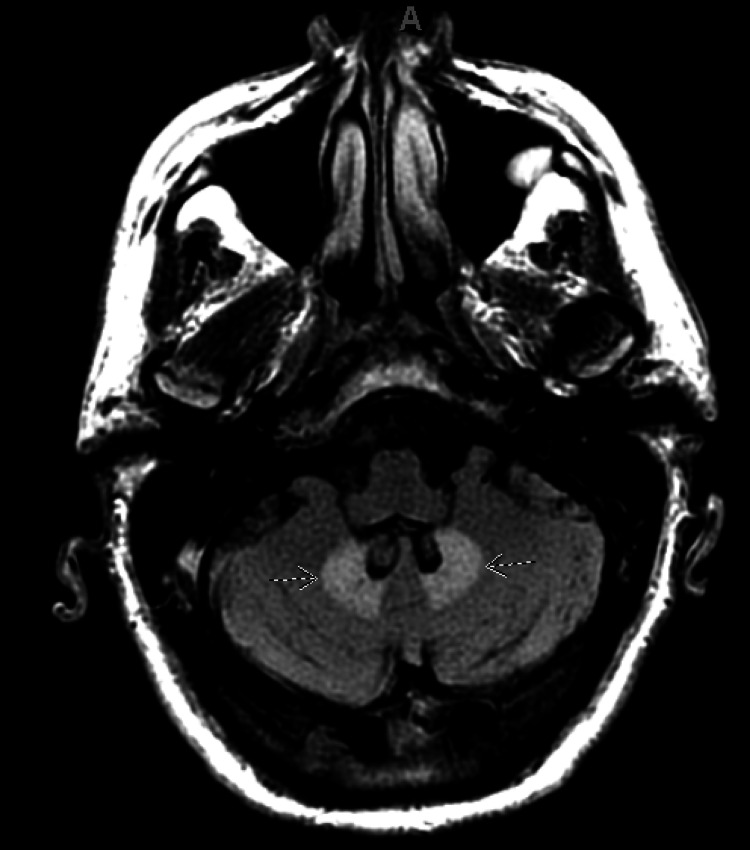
T2 FLAIR Brain MRI Without Contrast. Brain MRI of the patient revealed T2 FLAIR bilateral hyperintensity in the dentate nuclei of the cerebellum (arrows).
